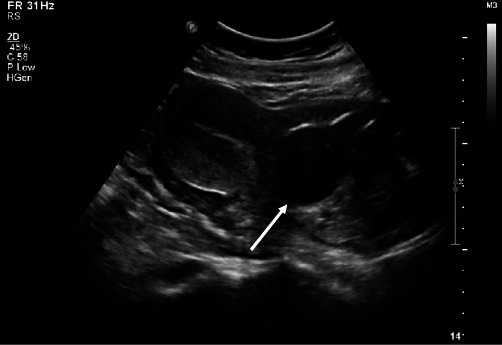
Transabdominal and sagittal ultrasound view demonstrating the Cook intracervical ripening balloon compressing the GS (arrow).
